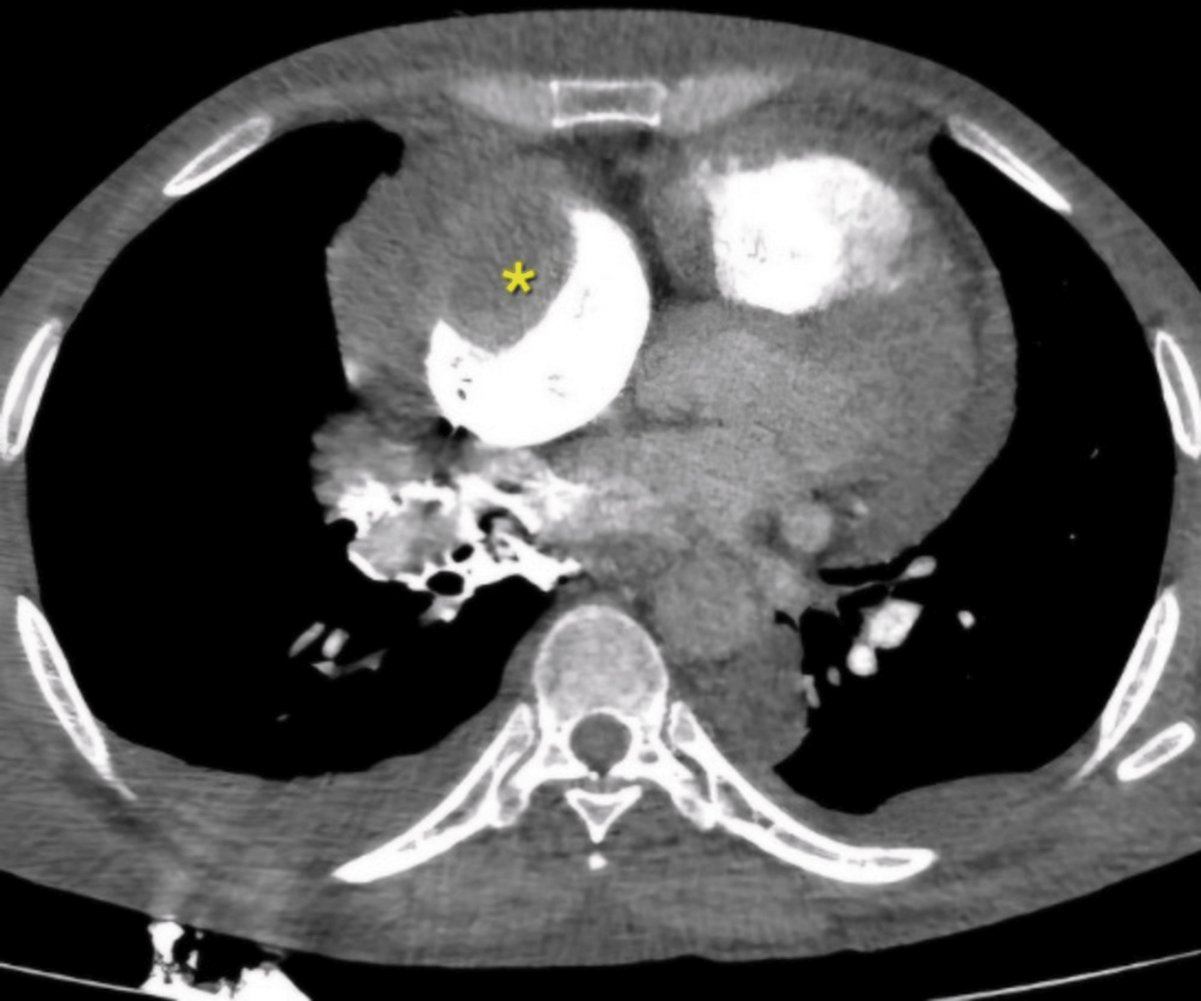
CT chest demonstrating right atrial thrombus (yellow asterisk)
